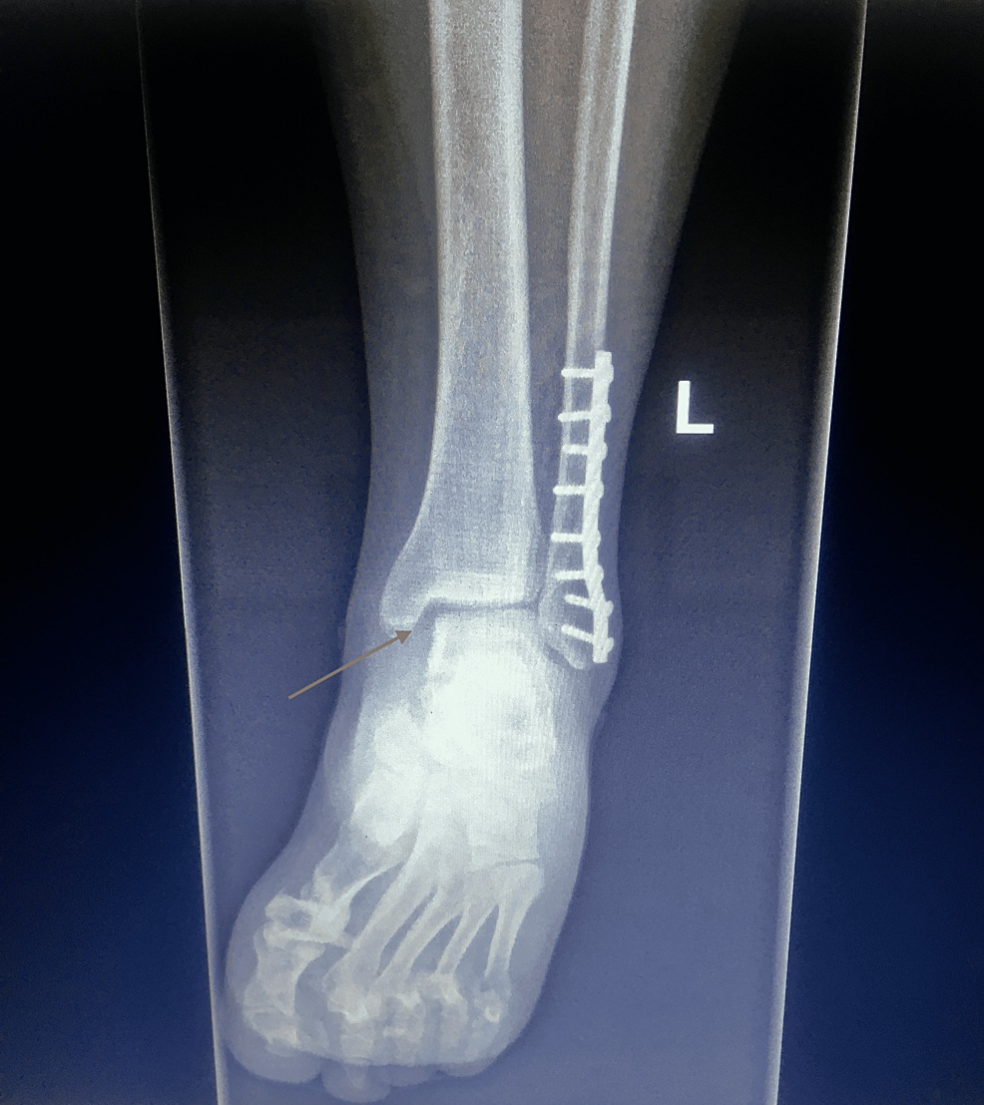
Preoperative roentgenography demonstrates an increased medial clear space and reduced tibiofibular overlap. No syndesmotic screw was used in the initial surgery.
